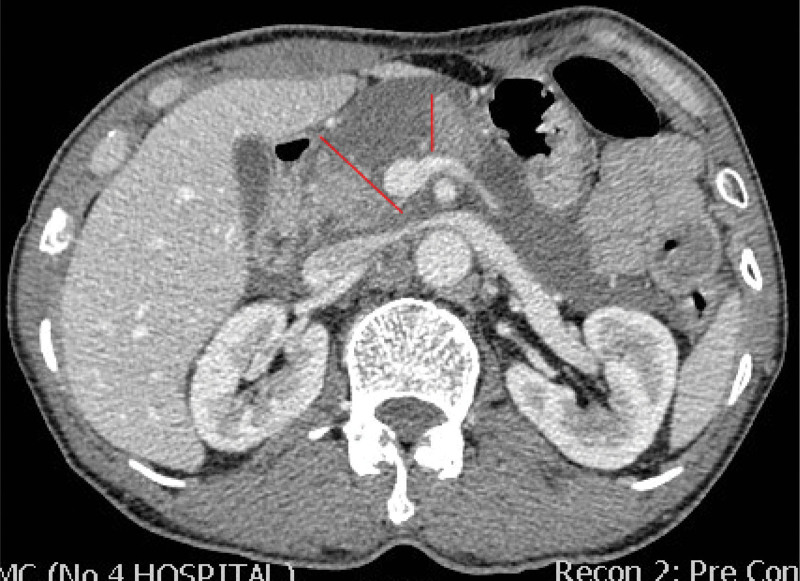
Emergency abdominal contrast-enhanced computed tomography showing transection (between red line) of pancreatic head and neck at the right of the superior mesenteric vein, suggestive of complete disruption of the MPD. MPD = main pancreatic duct.
